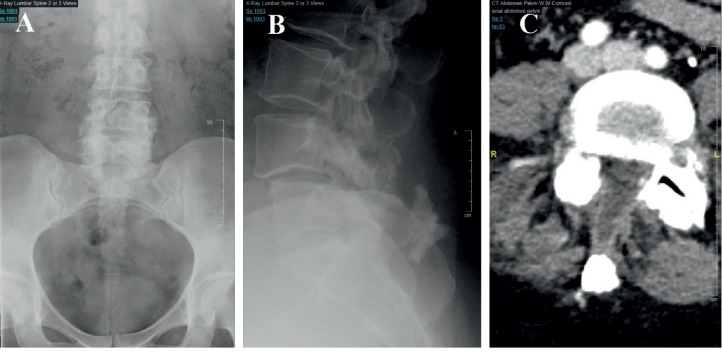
Preoperative X-ray and CT scan. (a) AP X-ray demonstrates the spina bifida occulta at L4-5; note the aberrant lamina. (b) Lateral X-ray demonstrates the L4-5 spondylolisthesis and the posterior subcutaneous calcification. (c) Axial CT image demonstrates the left L4-5 abnormal facet complex and the subcutaneous calcification.
